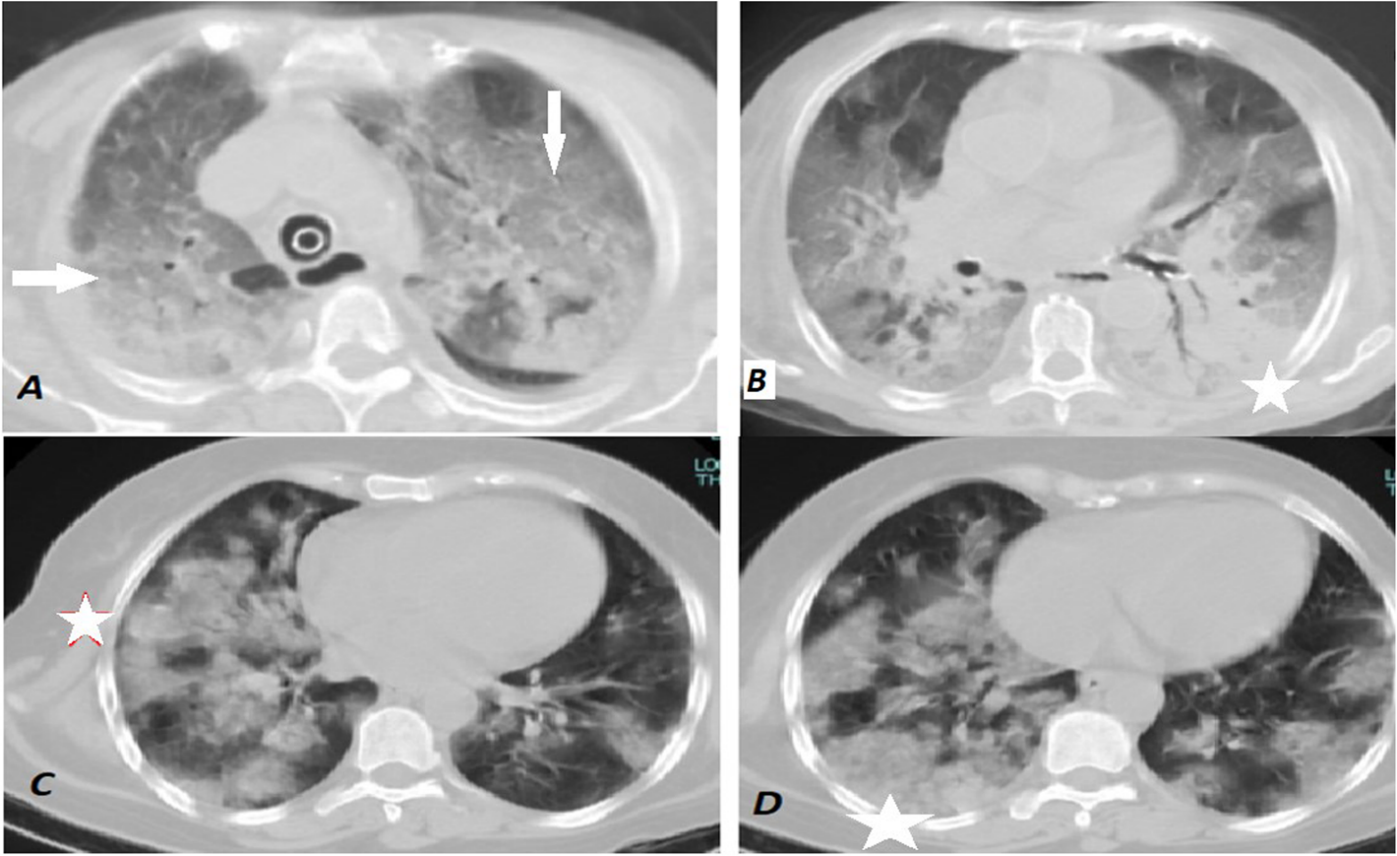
(A and B) Axial CT images, lung window, of a 63-year-old male who complained of persistent fever, cough, and severe dyspnea in the last 5 days. His images displayed almost bilateral diffuse ground-glass opacities (white arrows) with multiple consolidations mainly involving the left lower lobe (white star). The patient’s CT scan was reported as an intermediate probability for COVID-19 infection and PCR was positive and was admitted to ICU. His CT severity score was 20. (C and D) Axial CT images, lung window, of a 52-year-old male patient, who suffered from fever and severe dyspnea of 7 days duration and revealed bilateral lower lobar extensive predominant consolidation pattern (predominant consolidation CT phenotype) (white star). His RT-PCR tested positive for COVID-19 and received treatment in ICU. CT severity score was 18
